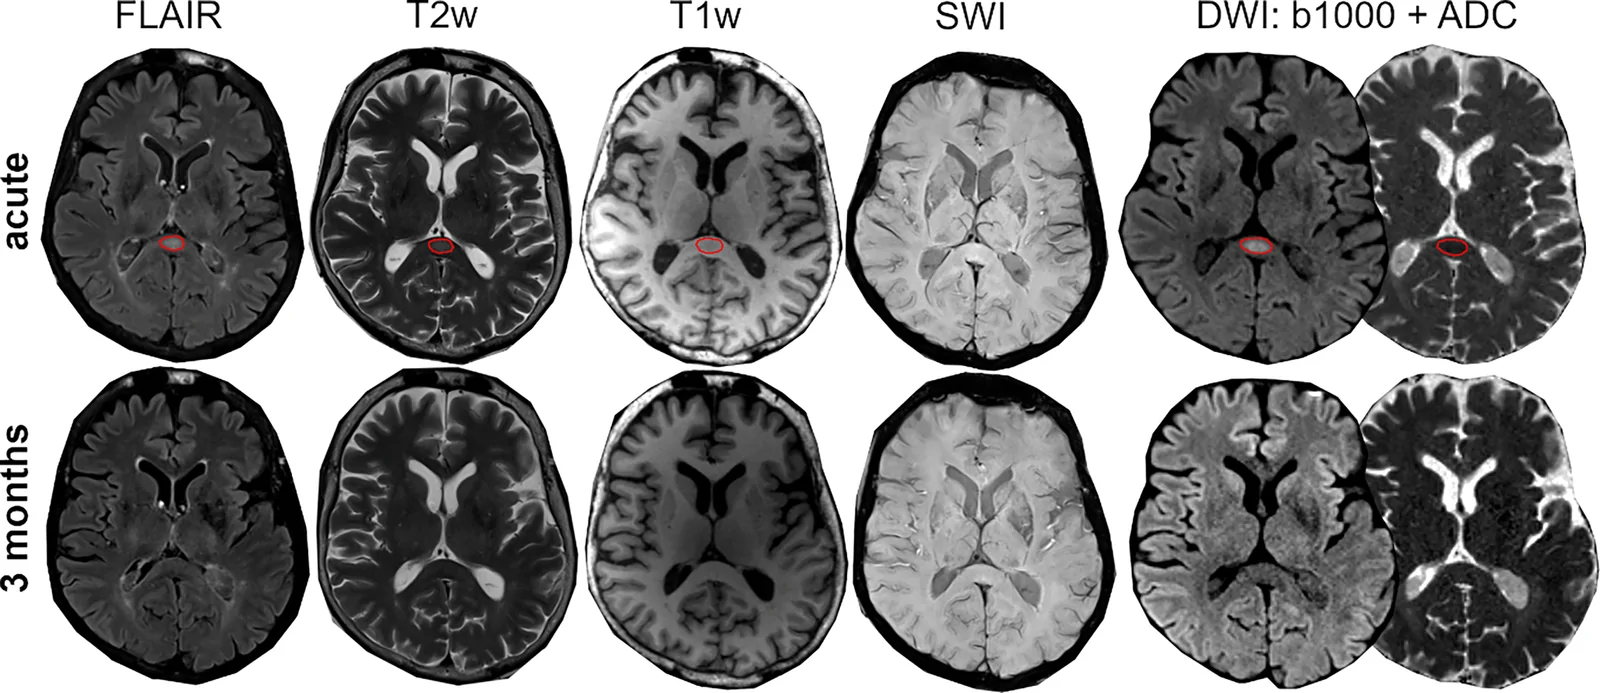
CLOCC on MRI. In the acute phase (top row) a typical FLAIR-/T2w-hyperintense, faintly T1w-hypointense diffusion restricted CLOCC was visible in the midline of the splenium (marked in red). CLOCC had completely resolved after 3 months (bottom row)
